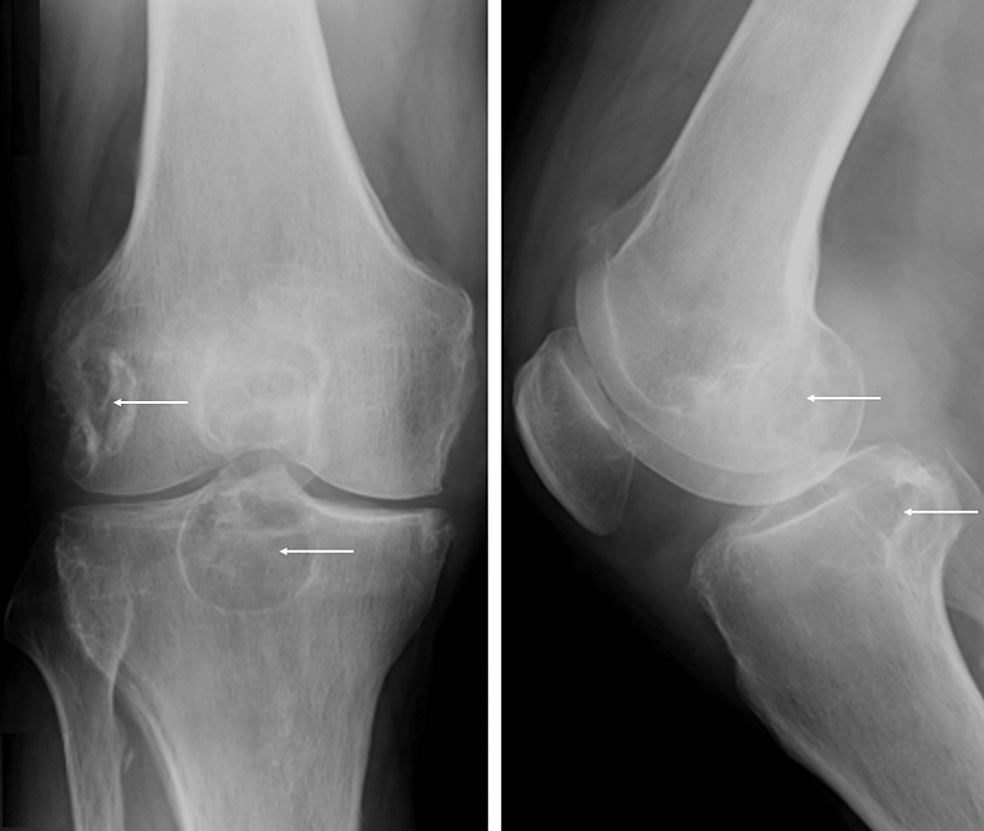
Radiographic images of the right knee Coronal view (A), sagittal view (B). The arrows point to the cystic formations in the proximal tibia and lateral distal femoral condyle
